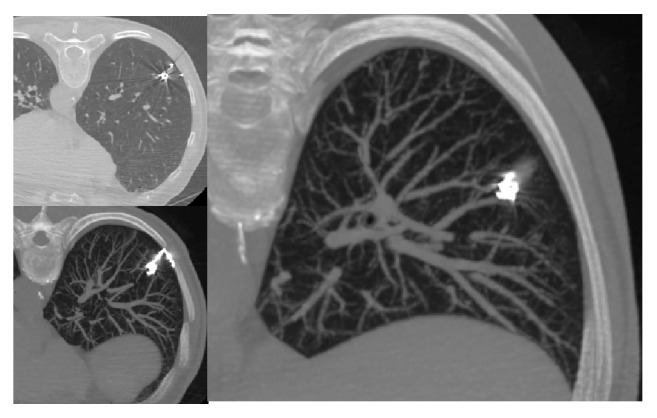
CT-images showing nodule localization by microcoil in different scanning planes.
